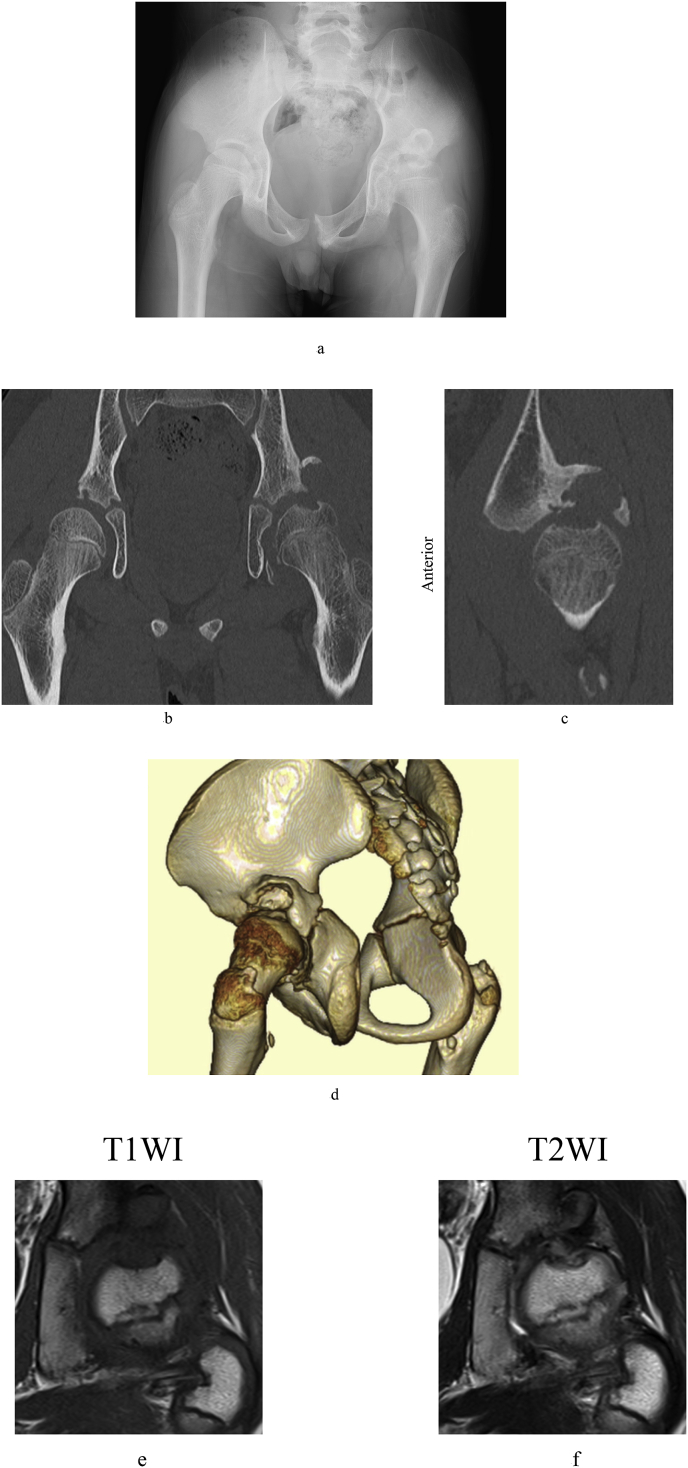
a) X-ray at 7 months after injury. Narrowing of the left hip joint space, deformity of the femoral head, narrowing of the obturator foramen, and the break in the Shenton line can be seen. b) CT coronal section at 7 months after injury. Bone fragments can be seen on the lateral side of acetabulum. A localized defect in the upper portion of the femoral head can also be seen. c) CT sagittal section showing that the posterior acetabular wall has displaced proximally. d) Three-dimensional CT showing that the posterior acetabular wall has displaced proximally. e) Low signal intensity is seen on T1-weighted MRI. f) T2-weighted MRI showing isointensity, with necrosis of the femoral head.
